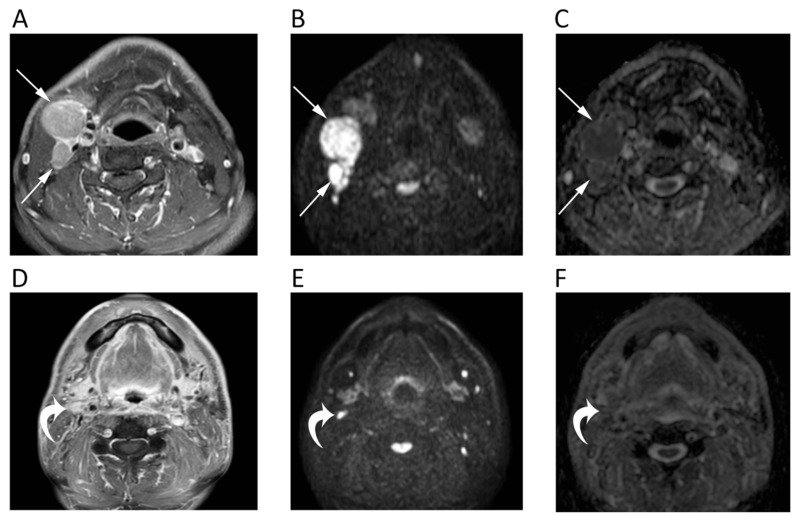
MRI scans of a 65-year-old male patient with oropharyngeal squamous cell carcinoma and lymph nodal metastases. Contrast-enhanced T1w (A), high *b*-value DWI (B), and corresponding ADC map (C) before chemotherapy show round enlarged right cervical lymph nodes (arrows) with restricted pattern of diffusion (mean ADC: 0.891 × 10^−3^ mm^2^/s). The same images after treatment (D–F) show the good response to chemotherapy with normal size and unrestricted pattern of diffusion of the cervical lymph nodes (curved arrows).
